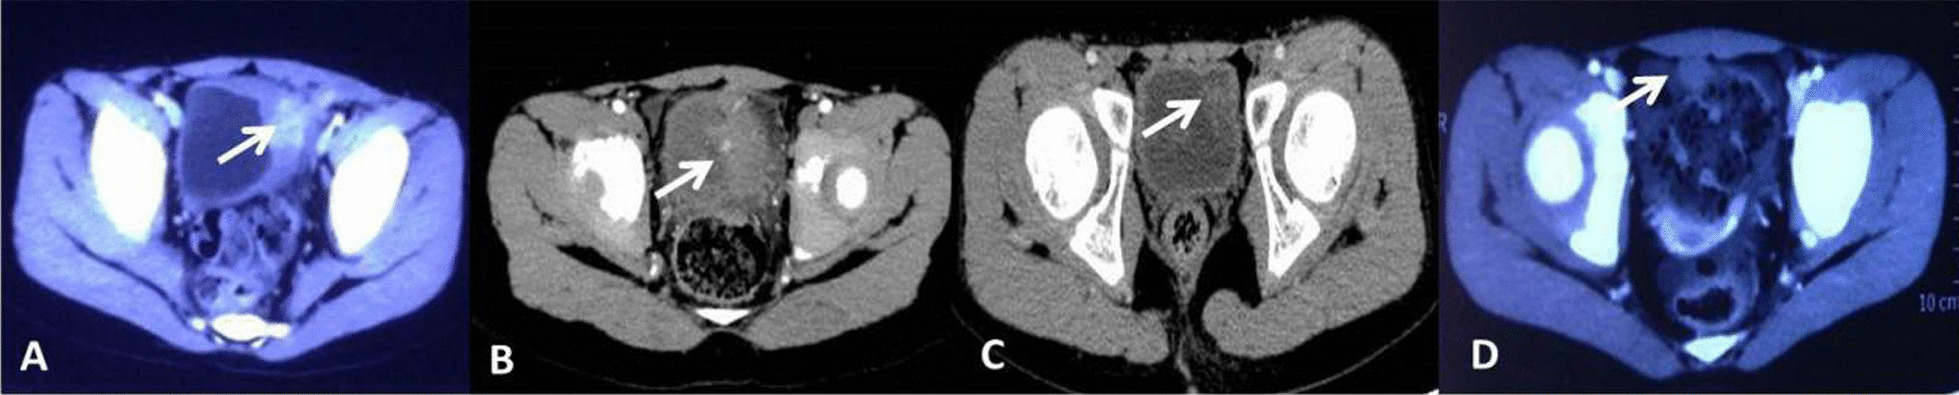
Photographs of CT scan: Patient 1 (a), Patient 2 (b), Patient 3 (c), Patient 4 (d); the arrow points to the tumor
